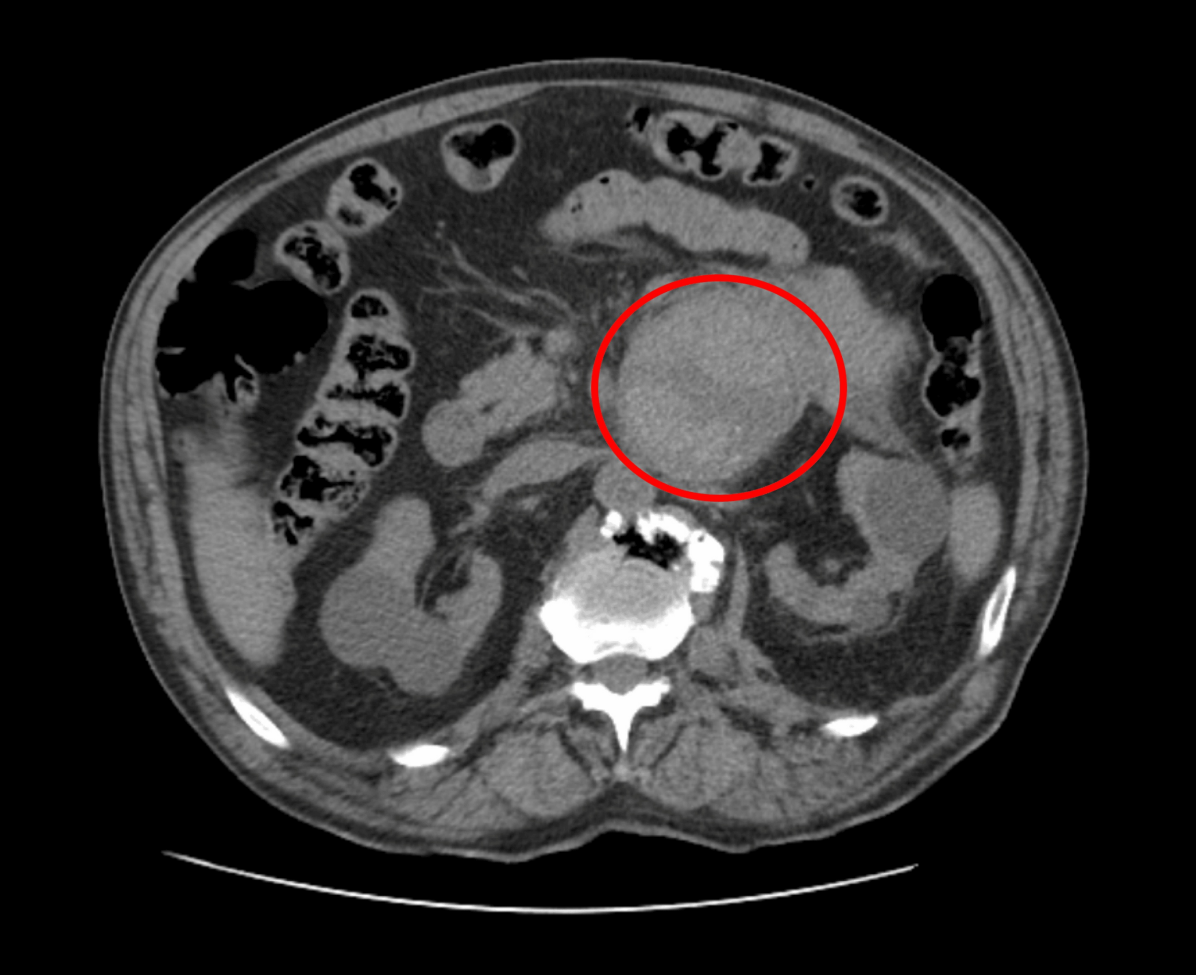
Abdominal computed tomography at admission, without contrast, showing the tumor (circle)
